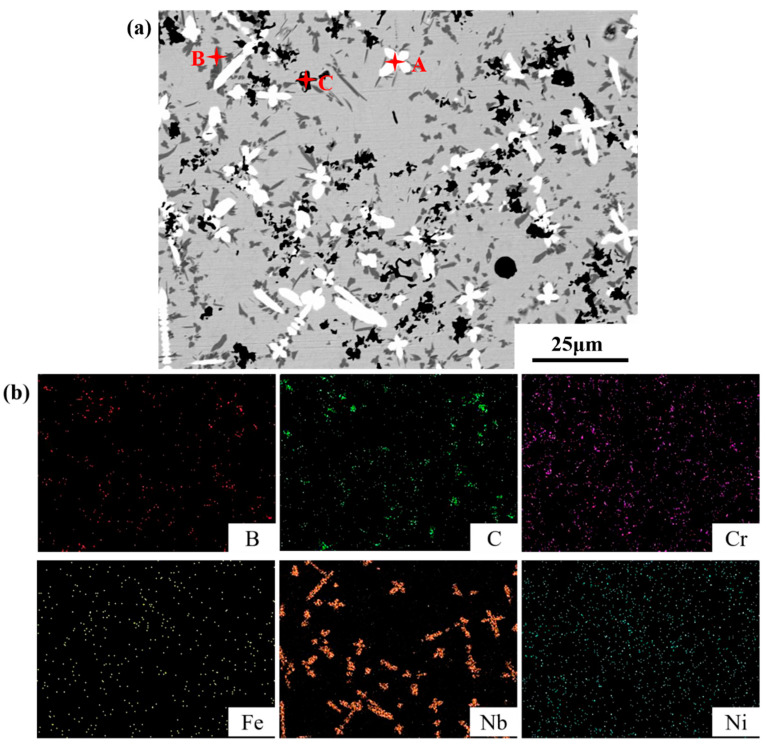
Microstructure (a) and element distribution (b) of coatings with ultrasonic vibrations.
